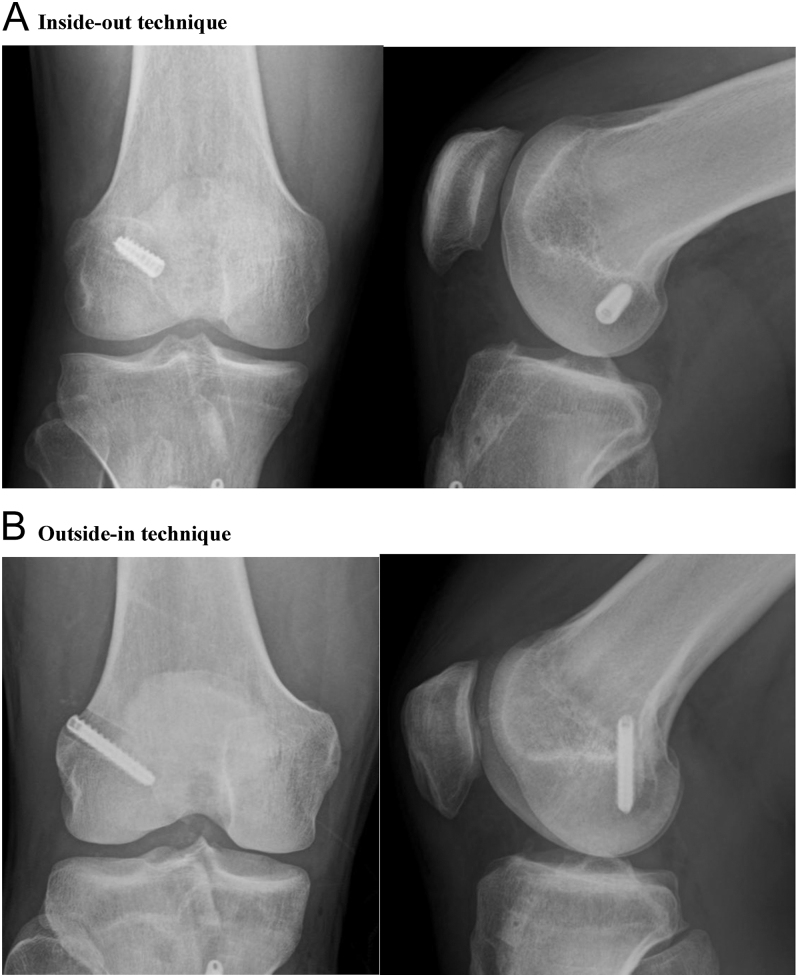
Plain X-ray with BTB fixed by metal interference screw: (A) inside-out technique and (B) outside-in technique.
